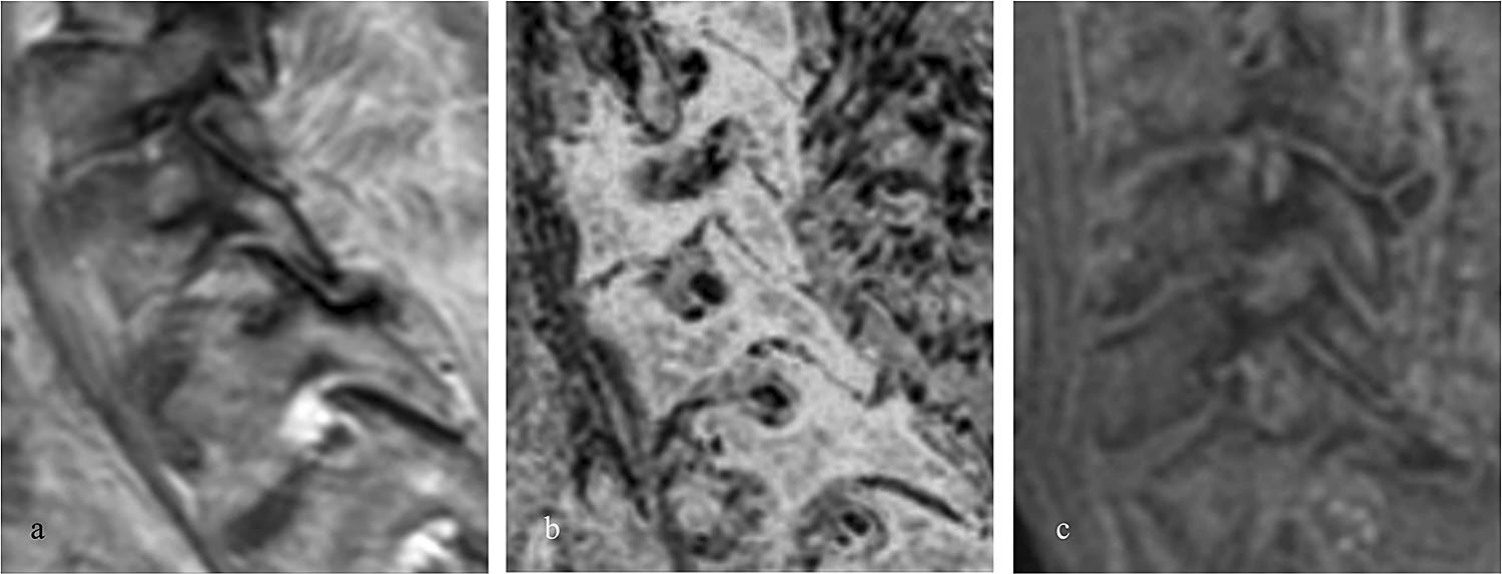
Images of neural foramina on T1-weighted TSE (a), FRACTURE (b) and UTE (c). Especially on UTE stenosis grading varied between readers, resulting in divergent changes of initially made diagnosis on standard MR sequences. On FRACTURE, both readers tended to upgrade stenosis gradings
